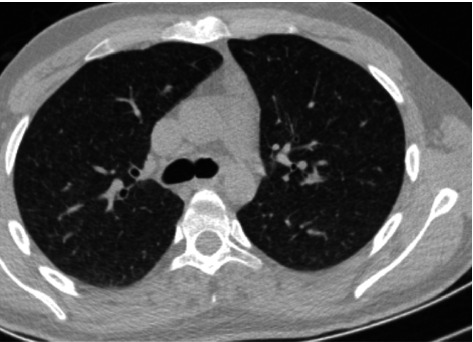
Thorax computed tomography (CT).
